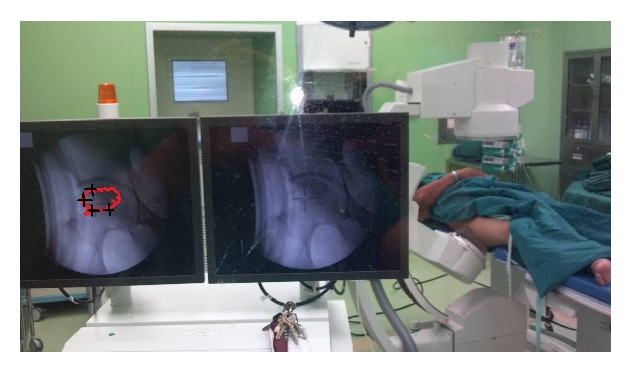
Image showing shockwave treatment of ONFH. Shock waves are applied under X-ray guidance. Four to six treatment points are located on the hardened layer around the necrosis lesion.
